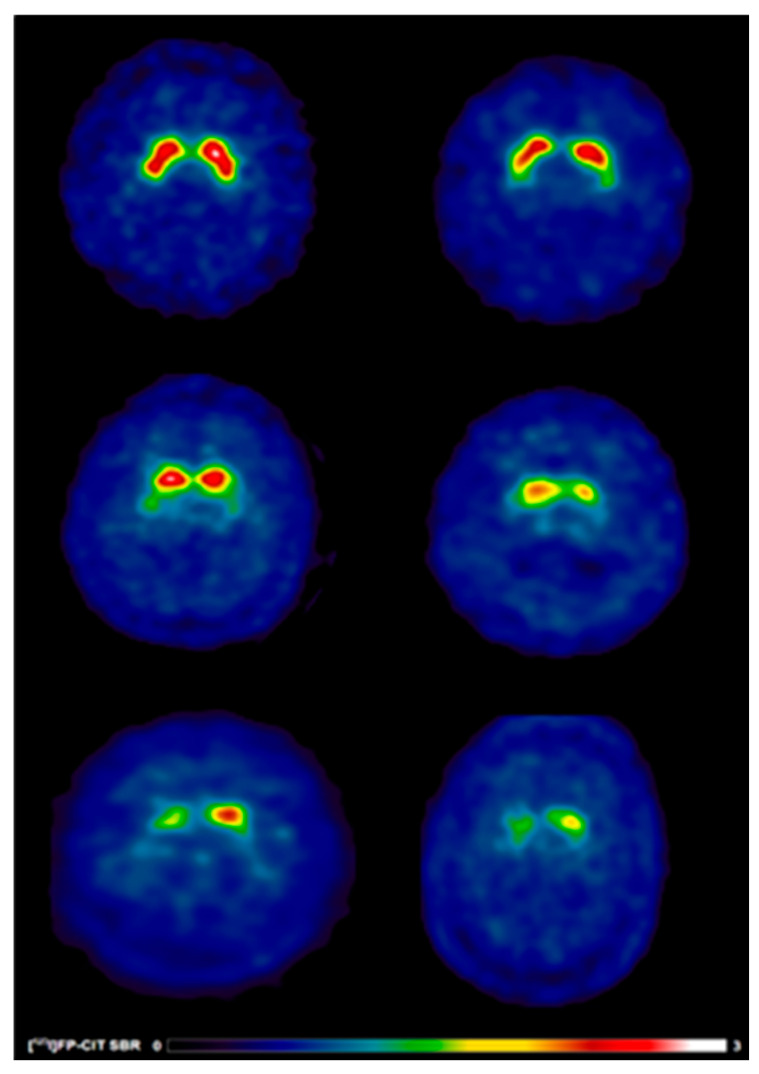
[^123^I]FP-CIT SPECT images in early treated PD patients with and without speech difficulties. Reduced [^123^I]FP-CIT binding reflects presynaptic nigrostriatal dopaminergic degeneration and should not be interpreted as an index of treatment responsiveness or pharmacological effect. Warmer colours indicate higher dopamine transporter availability, whereas cooler colours indicate reduced presynaptic dopaminergic binding. Anatomical regions of interest include the caudate nucleus and putamen bilaterally. (Top) A 65-year-old healthy control male (left) showing typical [^123^I]FP-CIT specific binding ratios in the caudate (SBR: 4.15) and putamen (SBR: 2.89) and a 69-year-old healthy control male (right) showing typical [^123^I]FP-CIT specific binding ratios in the caudate (SBR: 2.75) and putamen (SBR: 1.85). (Middle) A 65-year-old male (left) without speech difficulties exhibiting slight dopaminergic deficits as reflected by [^123^I]FP-CIT specific binding ratios in the caudate (SBR: 2.38) and putamen (SBR: 0.99) and a 69-year-old female (right) without speech difficulties exhibiting slight dopaminergic deficits as reflected by [^123^I]FP-CIT specific binding ratios in the caudate (SBR: 1.4) and putamen (SBR: 0.54). (Bottom) A 65-year-old male (left) with speech difficulties demonstrating larger striatal dopaminergic deficits as reflected by [^123^I]FP-CIT specific binding ratios in the caudate (SBR: 0.92) and putamen (SBR: 0.45) and a 69-year-old male (right) with speech difficulties demonstrating larger striatal dopaminergic deficits as reflected by [^123^I]FP-CIT specific binding ratios in the caudate (SBR: 1.36) and putamen (SBR: 0.71).
